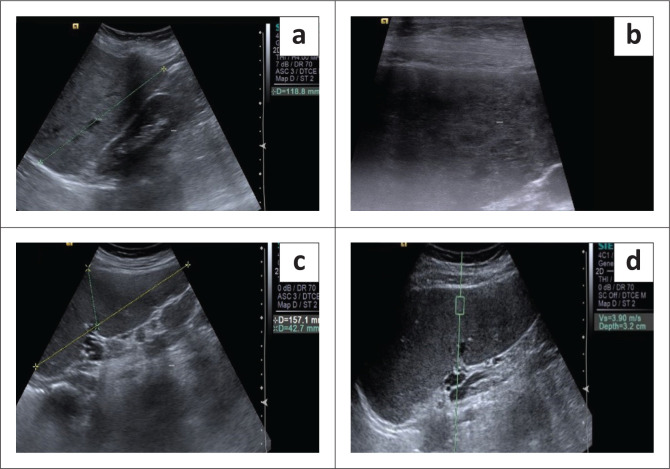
A 40-year-old female with chronic liver disease and oesophageal varices: Grey scale ultrasound reveals a normal sized liver with a heterogenous echo-pattern (a). Hepatic surface nodularity and echo-pattern is better appreciated with the high frequency probe (b). Splenomegaly with normal echo texture is seen (c). Acoustic radiation force imaging measurement taken in the splenic parenchyma is displayed in (d).
